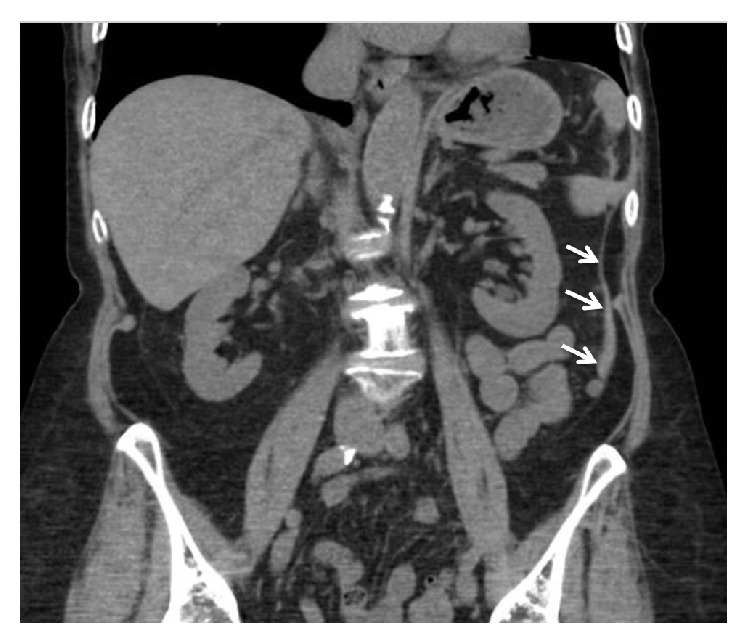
CT abdomen and pelvis without contrast. Coronal view showing small amount of high density perisplenic fluid (white arrows) tracking in the left paracolic gutter and extending into the pelvis.
